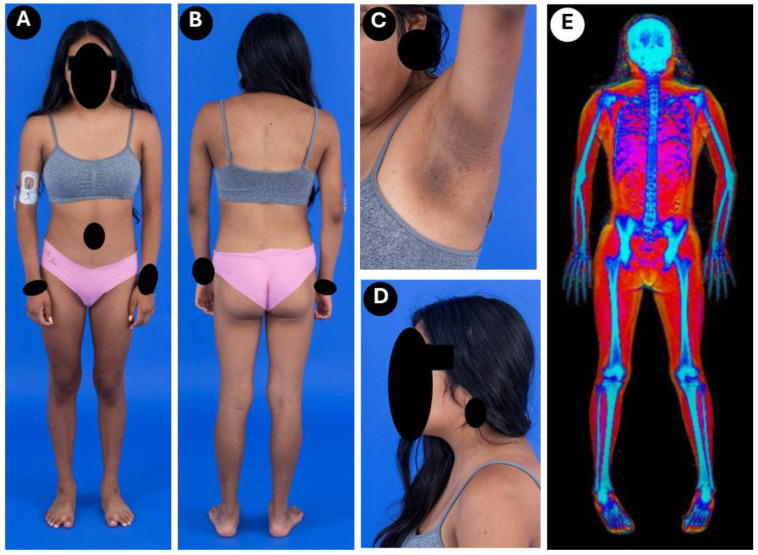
Phenotypic features and Dual-energy X-ray absorptiometry (DEXA) scan of the patient at the age of 15 years. (A) Anterior and (B) posterior view of the patient. Note decreased subcutaneous (sc) fat in the forearms, hips, and lower extremities, and preserved sc fat around the shoulders and upper arms. (C) Severe acanthosis nigricans in the axilla. (D) Increased adiposity in the neck and a small dorsocervical fat pad. (E) DEXA scan showing preserved fat around the shoulders and upper arms; however, sc fat in the forearms and lower extremities is markedly reduced. Total body fat by DEXA was 26.8% (−0.8 SD); regional fat in the upper extremities was 28.75% (−0.65 SD), and in the lower extremities was 24.1% (−2.3 SD). Her bone density measurements for the total whole body were 0.990 (z score 0.2), for the Lumbar region (L1 to L4) were 0.914 (z score 0.4), left femoral neck 0.789 (z score −0.1), total left hip 0.825 (z score −0.5), and at the left forearm were 0.64 (z score 0.5).
